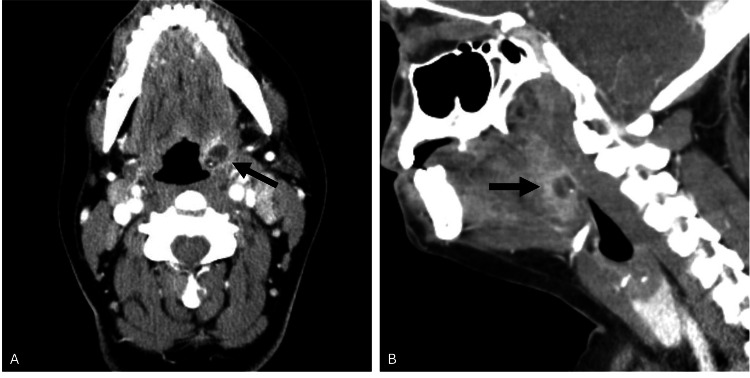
Computed tomography images of the neck Axial (A) and sagittal (B) contrast-enhanced CT scans of the neck demonstrate the presence of an isolated left ITA causing left palatine tonsil enlargement with central hypodensity and rim enhancement (black arrows). Septation is visualized as the hyperdense line within the left palatine tonsil. A small, circular, hyperdense calcification is visible in the inferior-posterior region of the left tonsil. ITA - intratonsillar abscess
